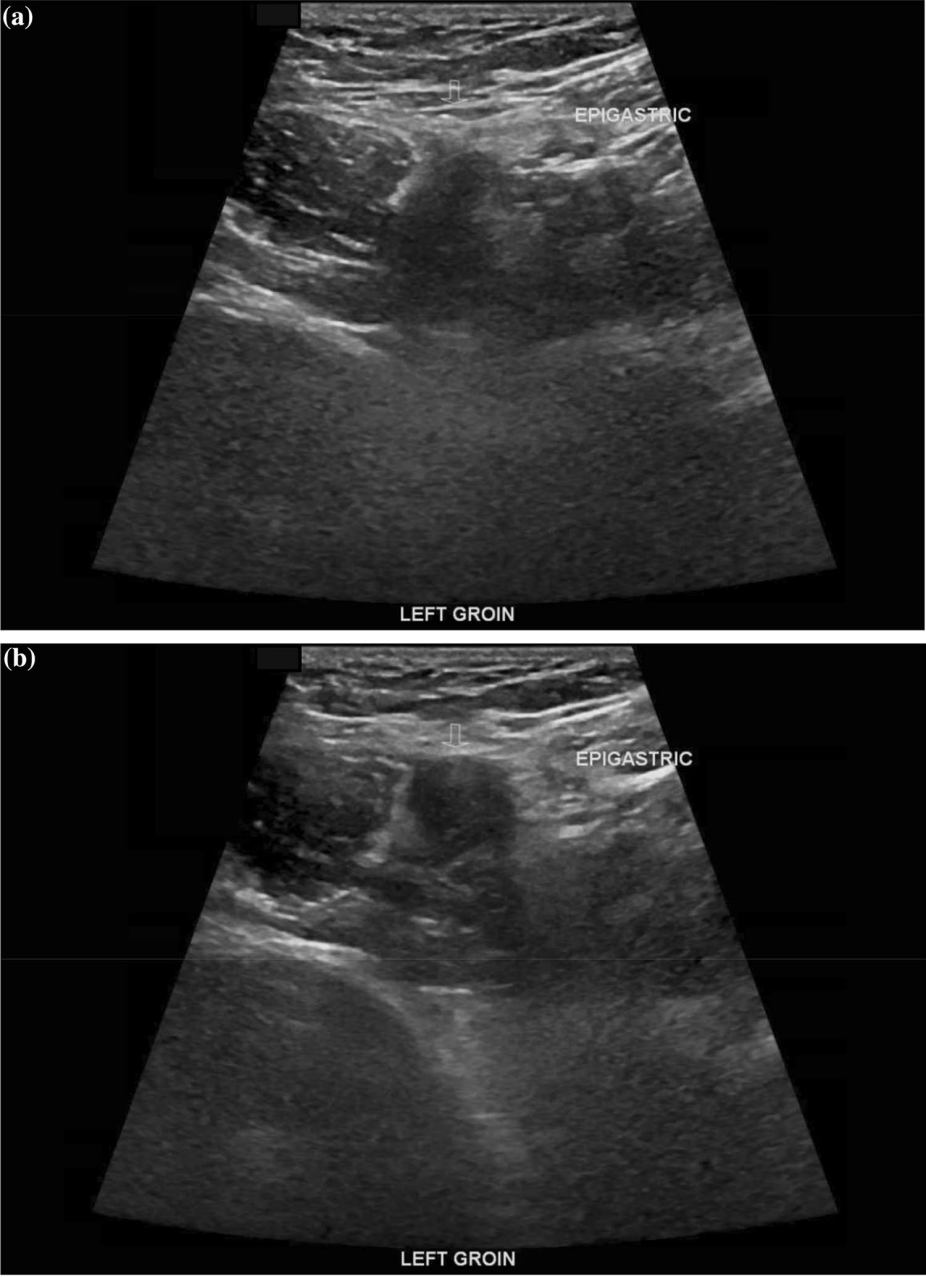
Ultrasound images demonstrating soft tissue protrusion medial to the epigastric vessels, labeled as epigastric in the image, within the inguinal canal in the left groin acquired pre-(a) and post (b)-valsalva. Open arrow indicates region of interest for soft tissue protrusion
